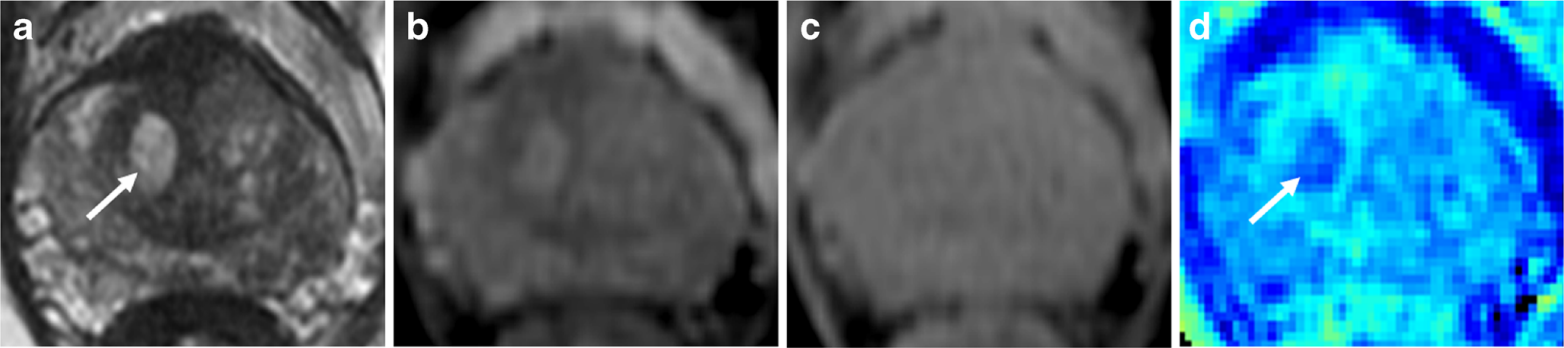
Cystic BPH demonstrated by magnetisation transfer imaging. A 67-year-old patient, PSA 6.39 ng/ml; target biopsy shows Gleason 3+4 tumour in the left mid TZ (not shown). T2-weighted imaging (a) shows a cystic area of BPH in the right apex transition zone (arrow); the area appears as high signal with MT “on” imaging (b), isointense on MT “off” imaging (c), and low signal on the magnetization transfer ratio image (d)
